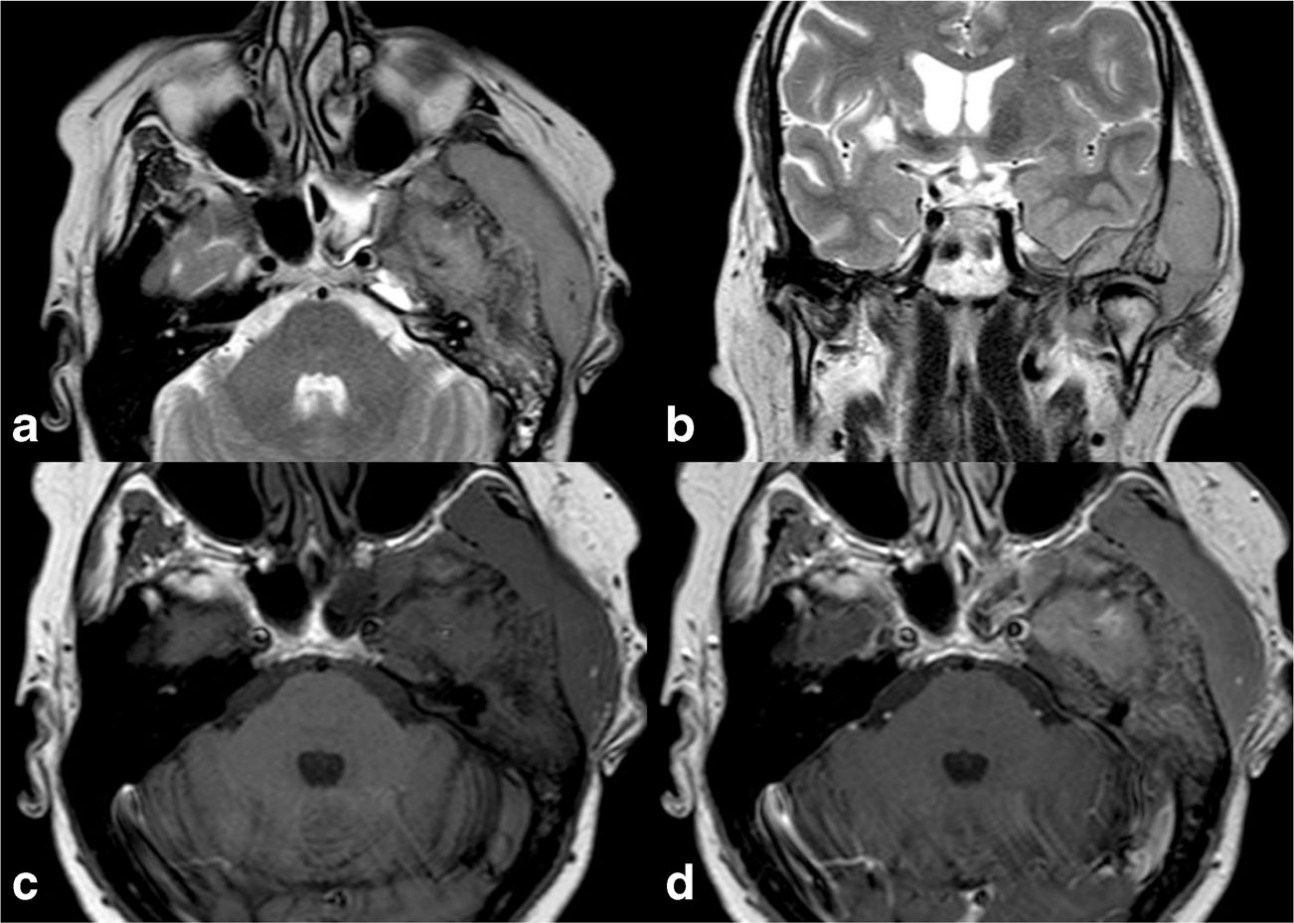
Primary lymphoma of the bone, same patient shown in Fig. 11. On MRI, the lesion demonstrates low T2 signal on axial (a) and coronal (b) T2-weighted sequences and extension to the temporal fossa and to the epidural space of the middle cranial fossa. The tumour exhibits homogeneous contrast enhancement; axial T1-weighted before (c) and after (d) contrast administration
